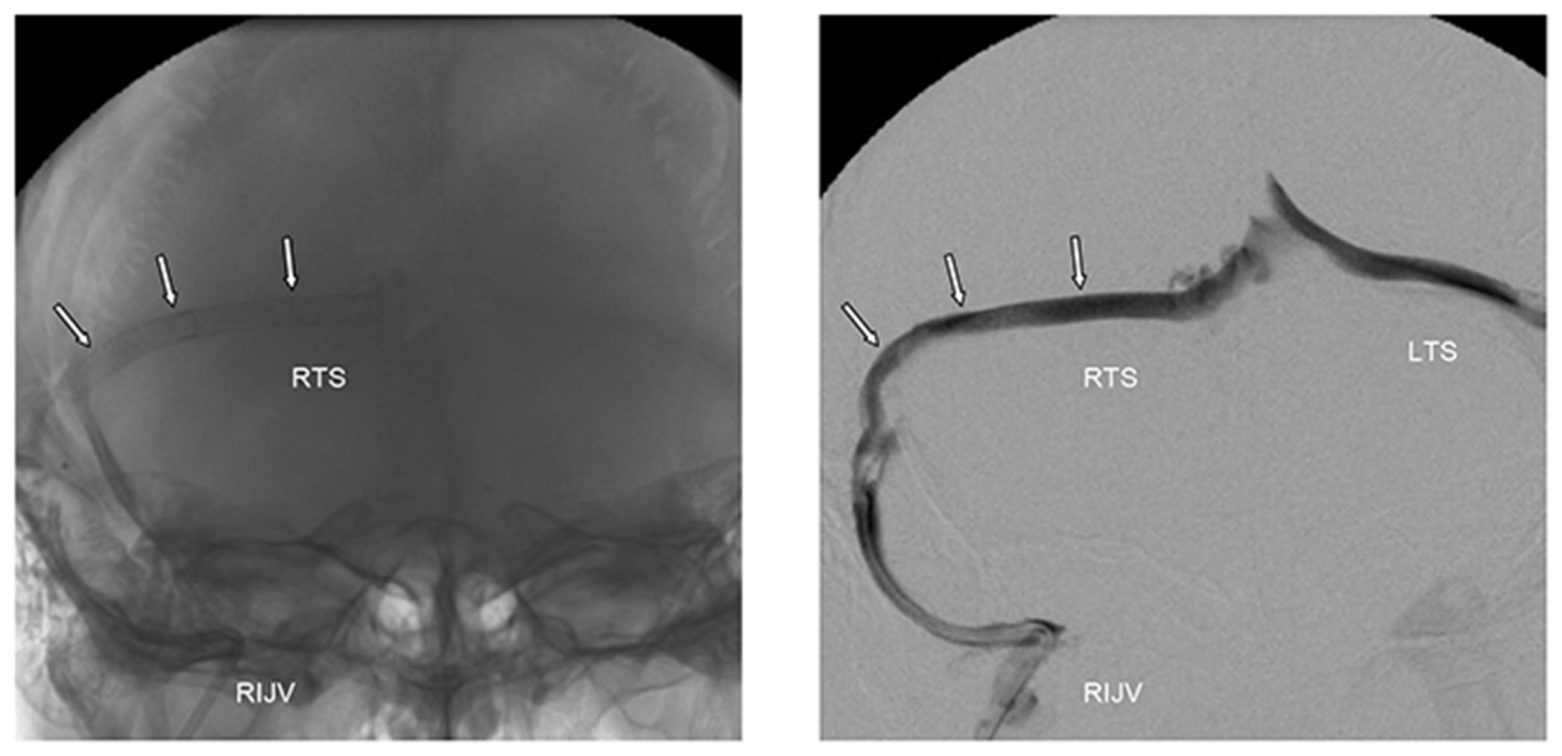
Frontal view venogram after stent placement in right transverse sinus Left image: unsubtracted film showing the stents along the right transverse sinus (arrows). The stent delivery catheter can be seen entering the sigmoid sinus from the right internal jugular vein. Right image: subtracted view in same projection following injection of the sinus with radiographic contrast. There is antegrade flow from the (stented) right transverse sinus (RTS) into the right jugular vein (RIJV) and retrograde flow across the confluence of sinuses into the left transverse sinus (LTS).
